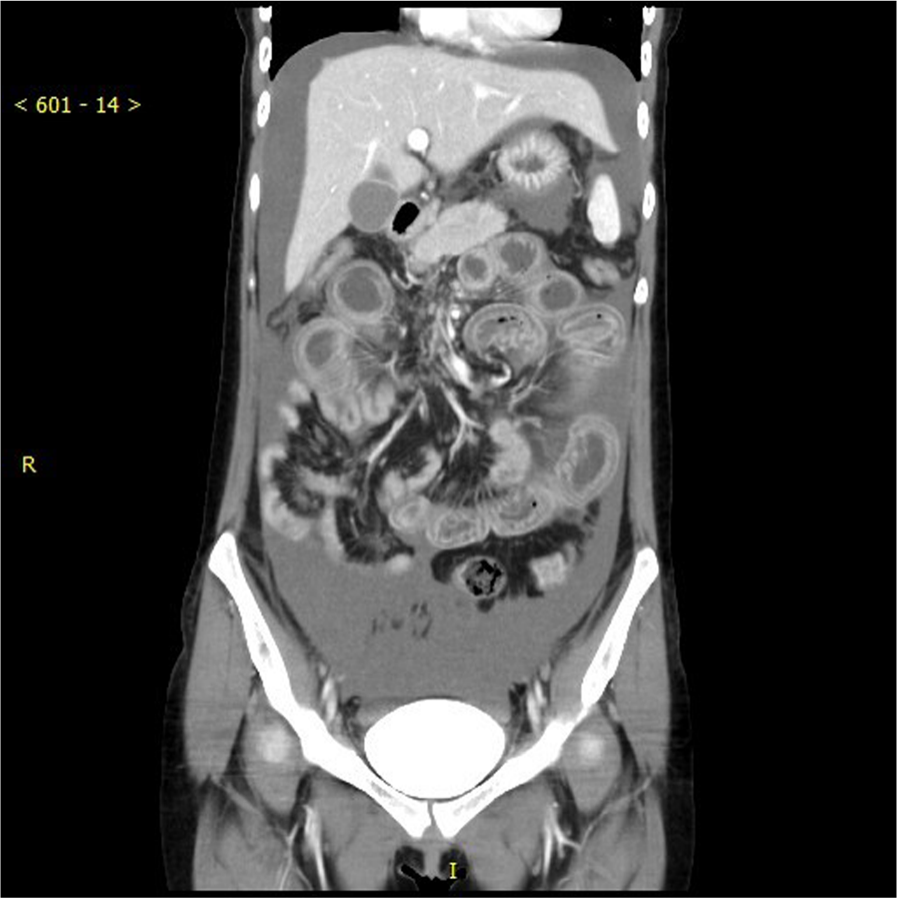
Coronary section of CT scan showing dilatation of proximal small intestine, massive ascites, no occlusion or filling defects in major mesenteric vessels
